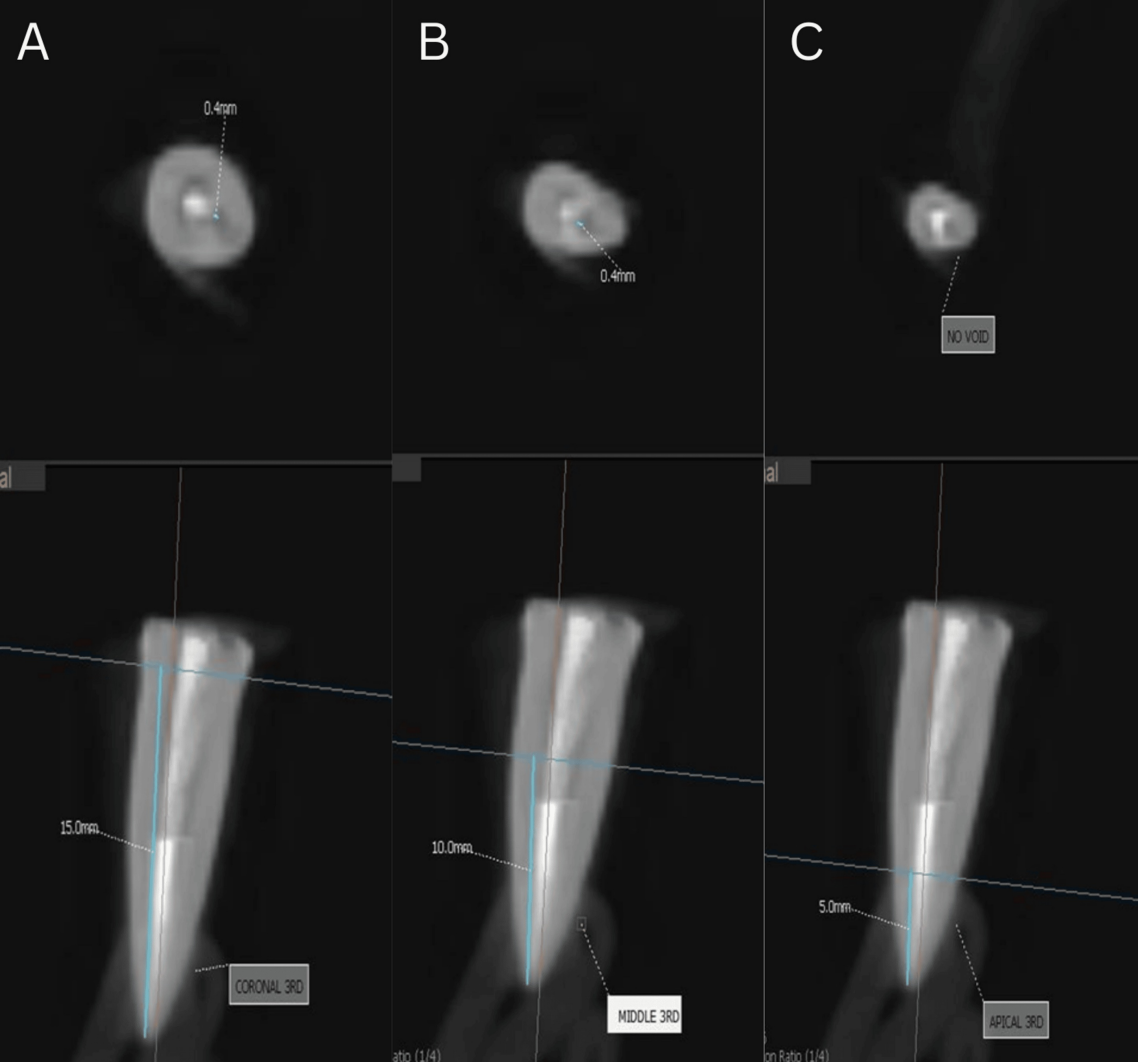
CBCT analysis of thermoplasticized gutta-percha technique CBCT images showing obturation with thermoplasticized gutta-percha technique in the (A) coronal third, (B) middle third, and (C) apical third.
